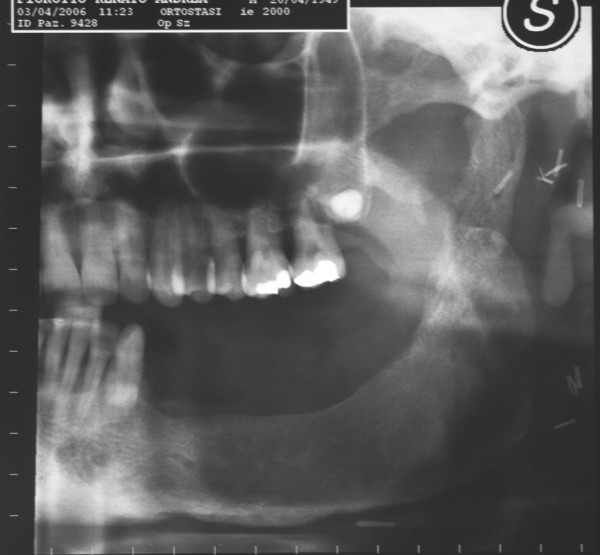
panoramic radiograph of patient n°2, 1 year after surgery.
